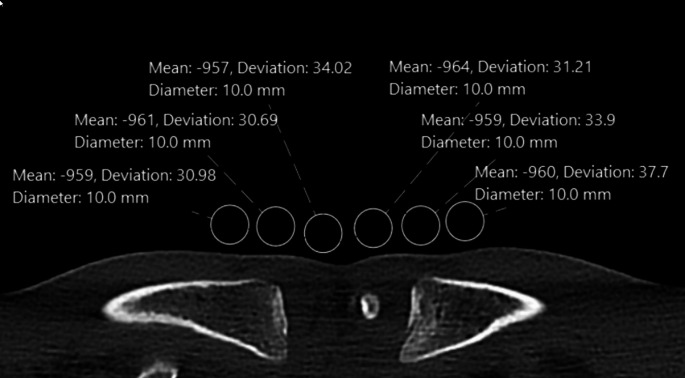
Noise measurements for air. To measure CT image noise in air, three 10 mm ROIs were placed in air, anteriorly to the right and left MCE at the midlevel of the clavicular epiphysis
